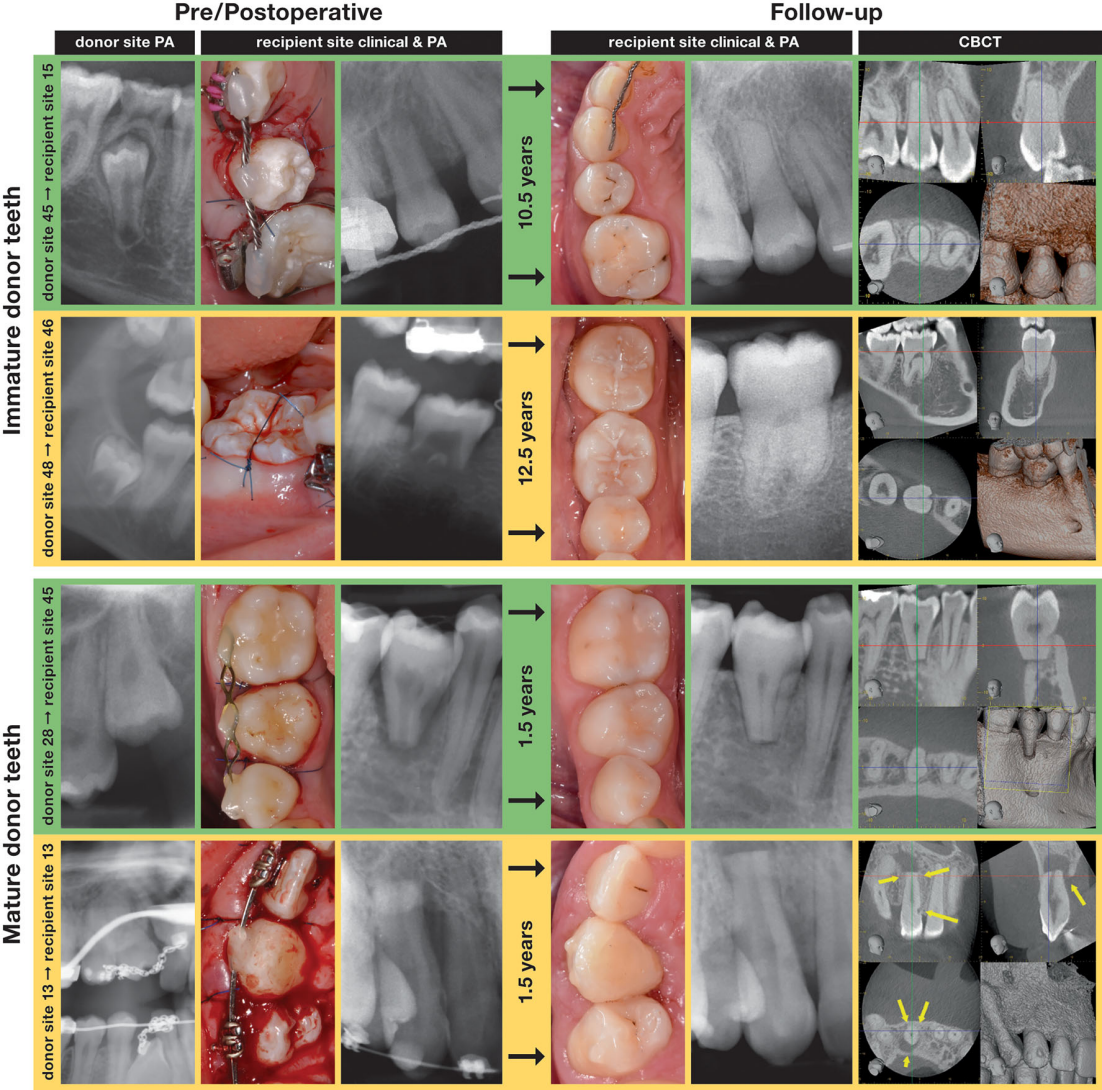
Four representative immature and mature transplants without (green) and with (yellow) postoperative potentially adverse findings. In the immature autotransplant, this finding was an intraradicular radiolucency at the level of the cementoenamel junction mesially at the transplant (i.e., invasive cervical resorption), which was only detectable with CBCT. In the mature autotransplant, the findings were discontinuous periodontal space, periapical radiolucency and an apico-marginal defect (i.e., replacement root resorption and apico-marginal lesion). Preoperative PA of the donor and recipient sites, postoperative clinical picture, and PA of the recipient site and CBCT, PA, and clinical picture at follow-up are shown
